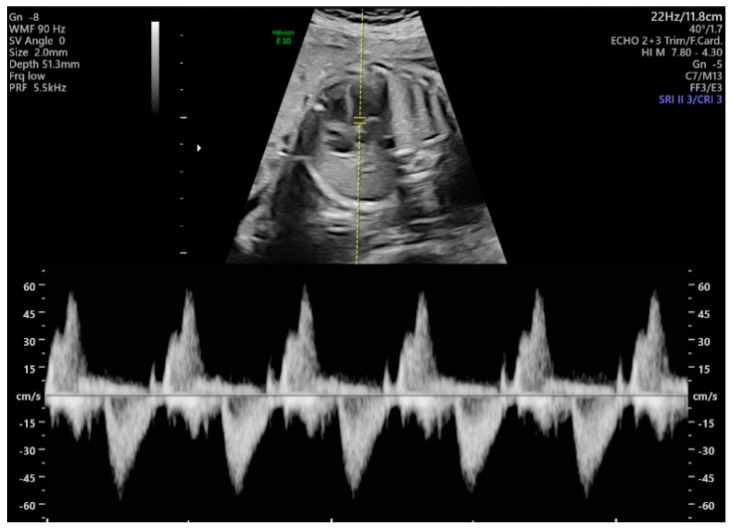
Two-dimensional (2D) images and Doppler waveforms of the measurements.
